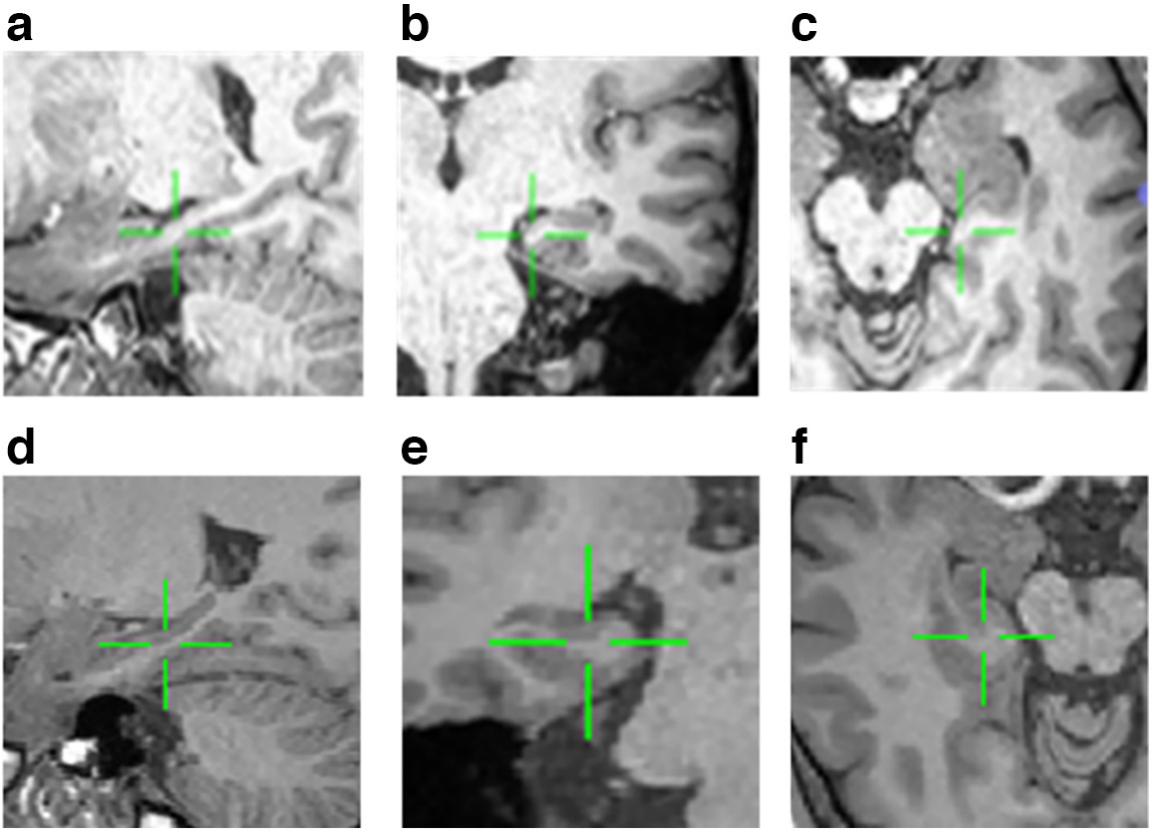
Electrode localization with structural magnetic resonance images. Examples of hippocampal microelectrodes from two patients are shown (see Table 1 for demographic information). The patient’s left brain is shown on the left side in the axis plane. All images are T1 images recorded using Sigma 3-tesla scanner (GE). Green arrows indicate electrodes inserted in the hippocampal subfield of Cornu Ammonis (CA3 (above) and CA1 (bottom)). *a*, Sagittal image of the left hippocampal CA3. *b*, Coronal hippocampal CA3. *c*, Axial hippocampal CA3. *d*, Sagittal image of the left hippocampal CA1. *e*, Coronal CA1 image. *f*, Axial CA1 image.
